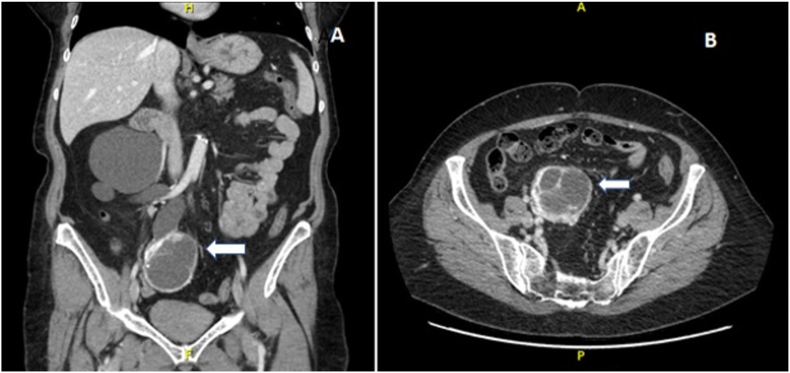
A. CT abdomen with contrast, coronal view. B. CT of the abdomen with contrast, axial view. Redemonstration of severe right hydronephrosis and hydroureter down to the level of the mid ureter, secondary to obstruction from what appeared to be a right ovarian mass.
